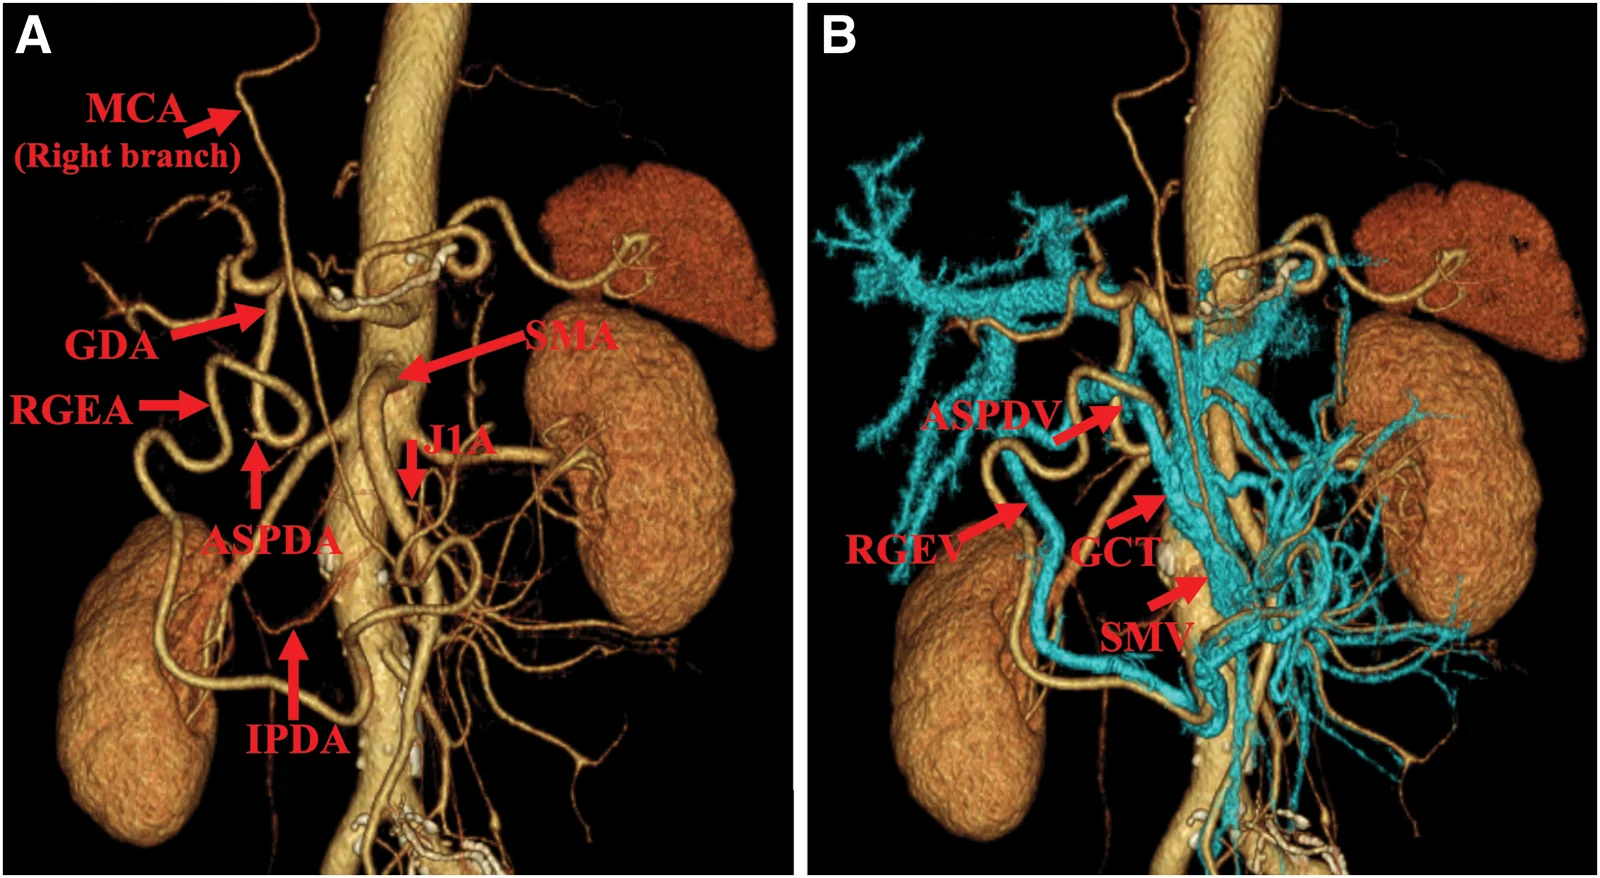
Preoperative vascular anatomy relevant to surgery. (A) 3D-reconstructed CT showing the arterial anatomy, including the GDA, ASPDA, RGEA, SMA, IPDA, J1A, and the right branch of the MCA. The bifurcation of the ASPDA and RGEA from the GDA was clearly identified preoperatively. (B) 3D-reconstructed CT showing the venous anatomy, including the RGEV, ASPDV, SMV, and GCT. ASPDA, anterior superior pancreaticoduodenal artery; ASPDV, anterior superior pancreaticoduodenal vein; GCT, gastrocolic trunk; GDA, gastroduodenal artery; IPDA, inferior pancreaticoduodenal artery; J1A, first jejunal artery; MCA, middle colic artery; RGEA, right gastroepiploic artery; RGEV, right gastroepiploic vein; SMA, superior mesenteric artery; SMV, superior mesenteric vein
